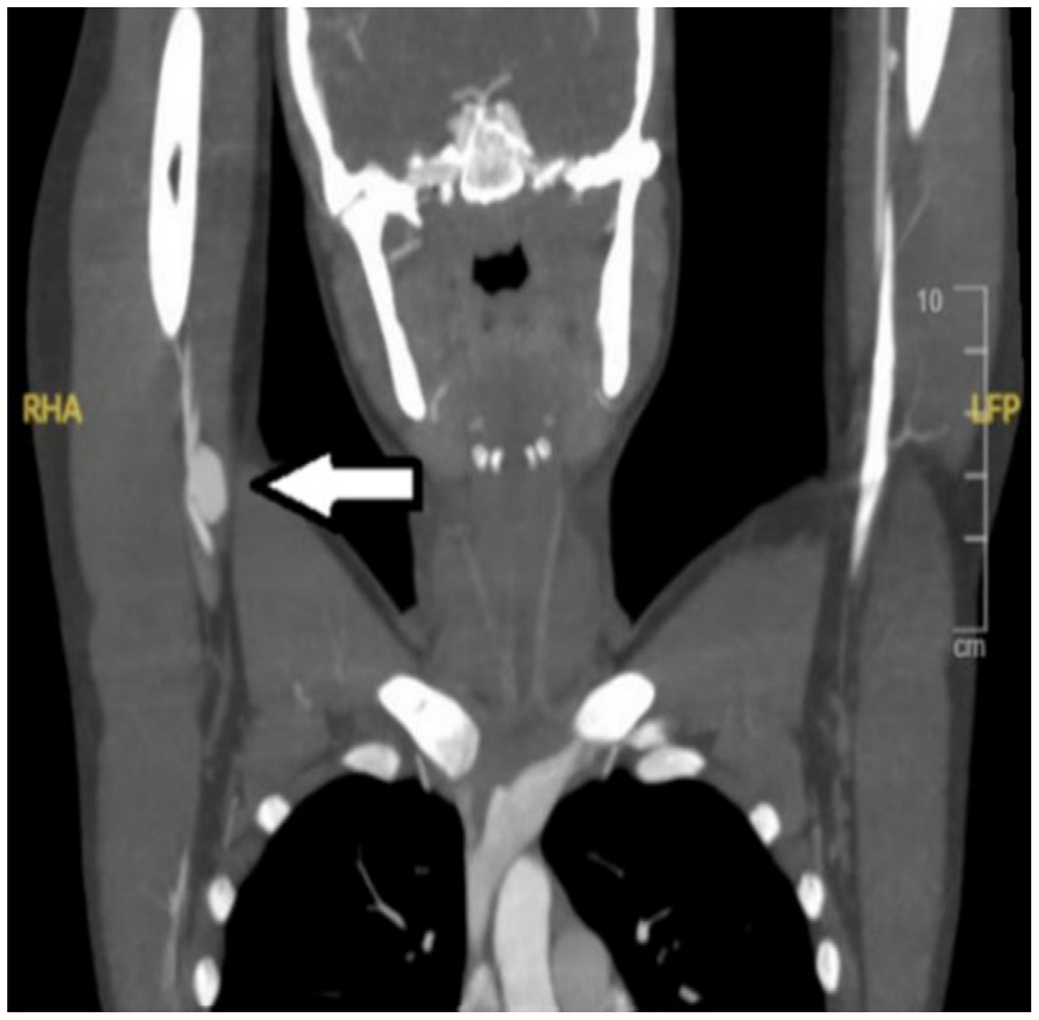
Computed tomography arteriogram demonstrating right axillary artery aneurysm.
